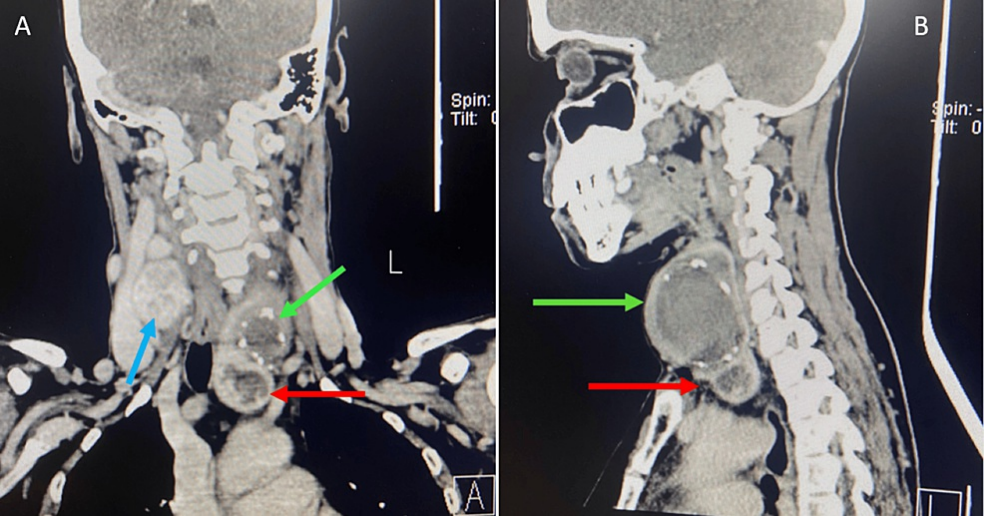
Contrast-enhanced computed tomography (CECT) scan of the head and neck showing a biloculated cystic lesion in the left lobe with calcification (shown in green arrow in A & B) and retrosternal extension into superior mediastinum (shown in red arrow in A & B). The right lobe contained a cystic lesion with multiple foci of calcifications (shown in blue arrow in A). A on the left shows the coronal view, and B on the right shows the sagittal view
